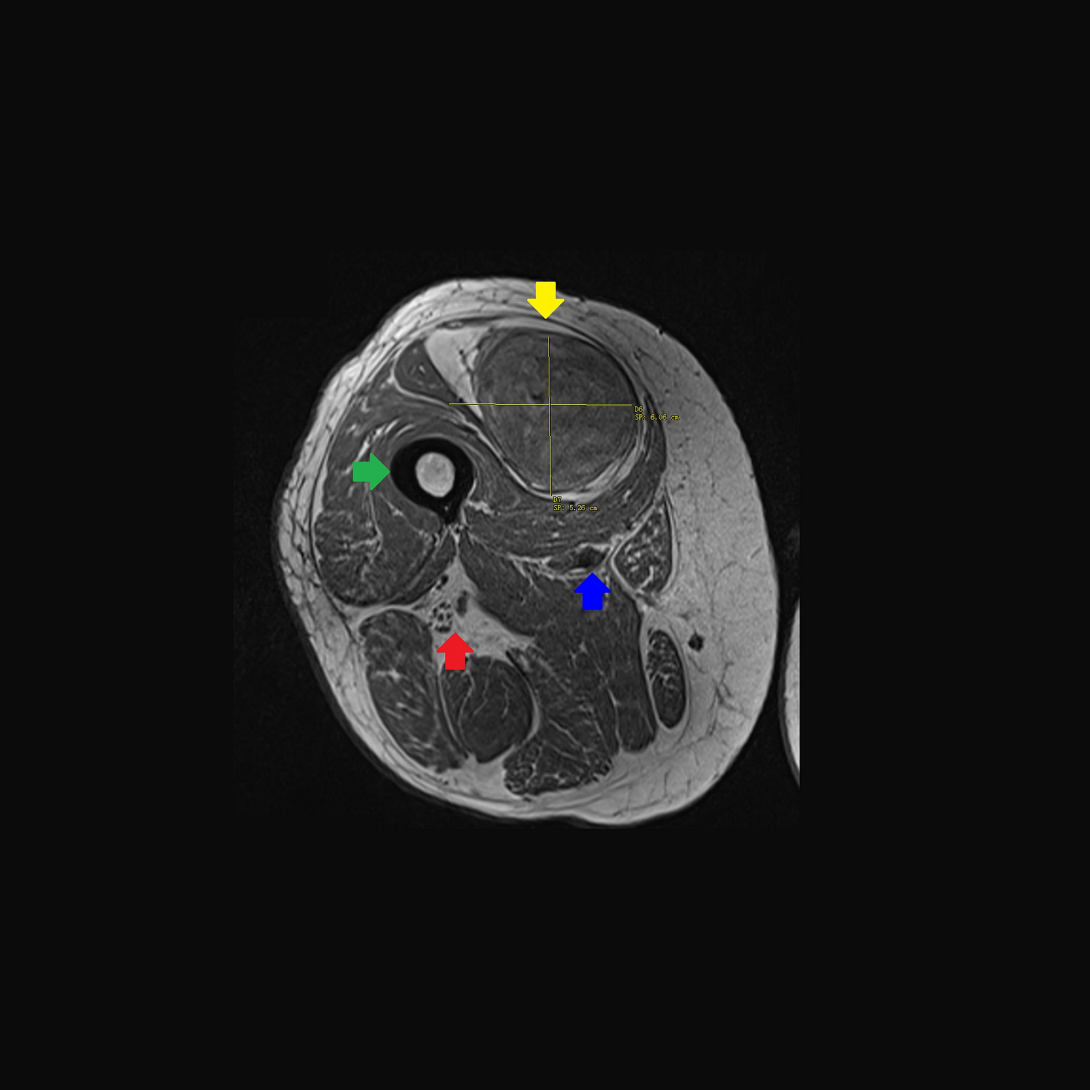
T1-weighted turbo spin echo MRI without contrast of the right thigh in axial plane. A 20 × 6 × 5 cm multi-loculated mass (yellow arrow) with a superior lipogenic component and inferior non-lipogenic component (9 × 5 × 5 cm). There is no invasion of the femur (green arrow), profunda femoris artery/vein (red arrow), or superficial femoral artery/vein and posterior division of the femoral nerve (blue arrow). The tumor displaces the rectus femoris medially and the vastus intermedius posteriorly. MRI, magnetic resonance imaging
